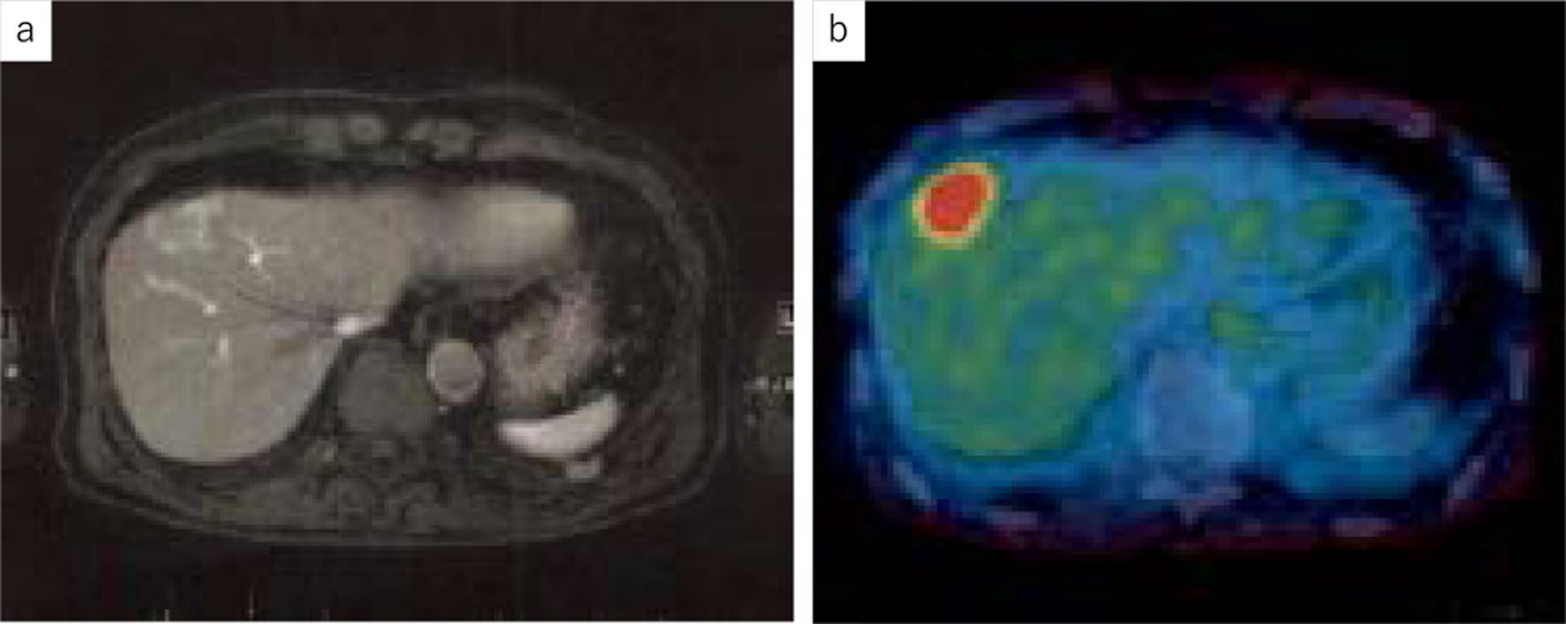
a Abdominal computed tomography scan showed a 40-mm solitary tumor in segment 4 of the liver. b Fluorodeoxyglucose positron emission tomography (FDG-PET) showed FDG uptake by the liver tumor
